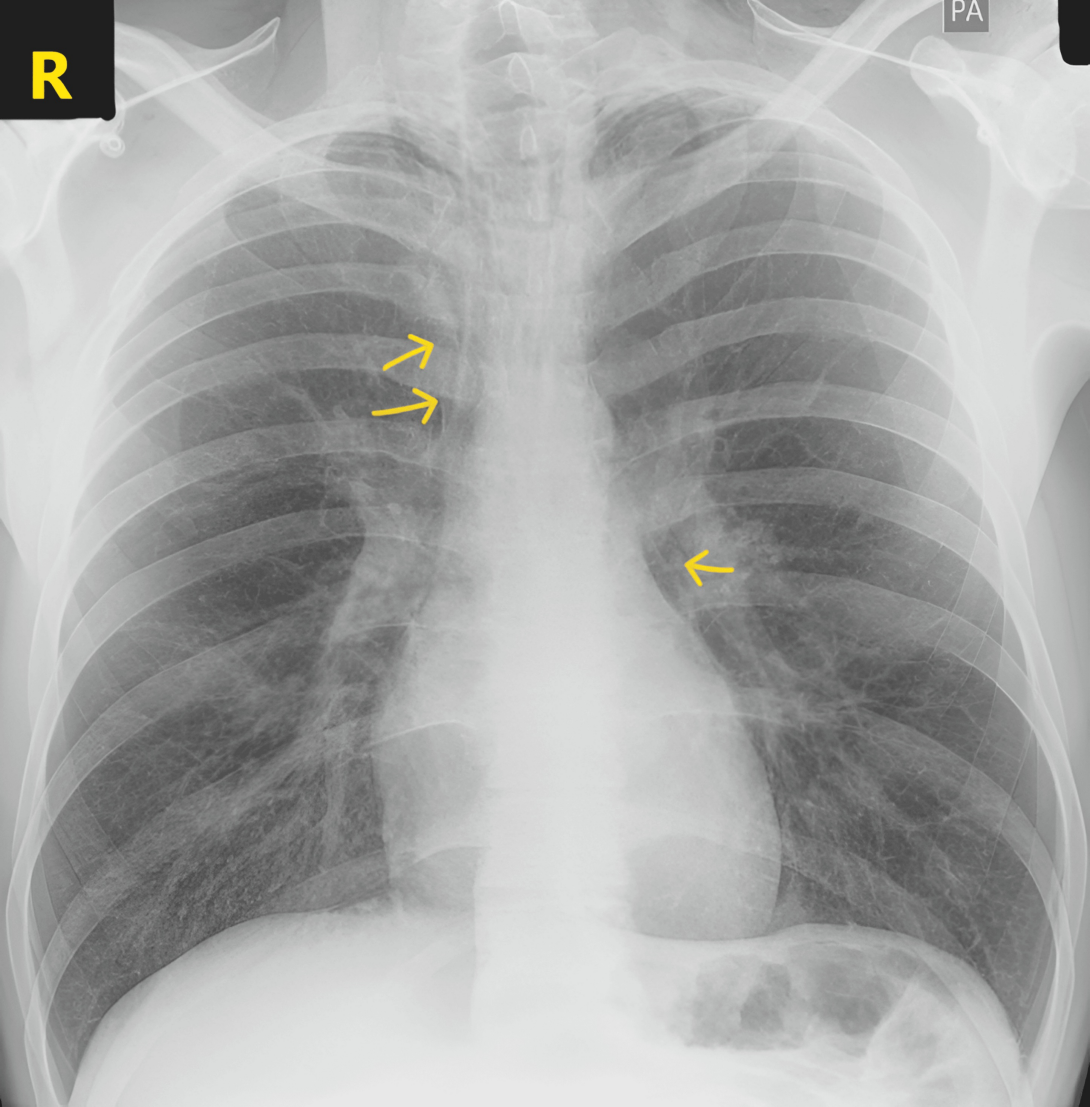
Chest X-ray (posteroanterior view) showing pneumomediastinum (yellow arrow).
